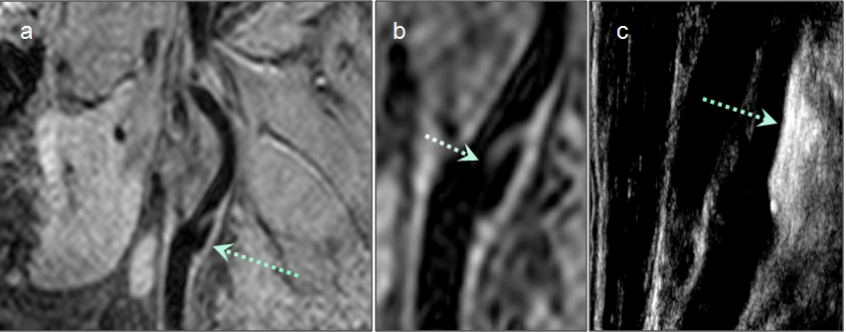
Original CMR slice data cropped (a) and zoomed to lesion, indicated by arrows (b). The ultrasound image (9 MHz) at the according position, showing the severely calcified lesion in the left carotid artery bifurcation, is depicted in (c). Images correspond to the patient depicted on the right in Fig. 2.
